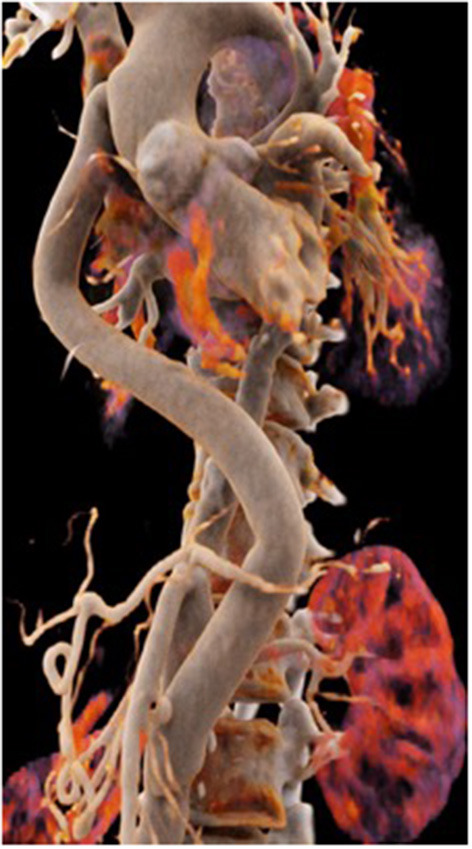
Postoperative 3-D reconstruction of the CT-angio following ascending to infrarenal bypass in the patient with very calcified and narrowed descending aorta following Takayasu disease.
